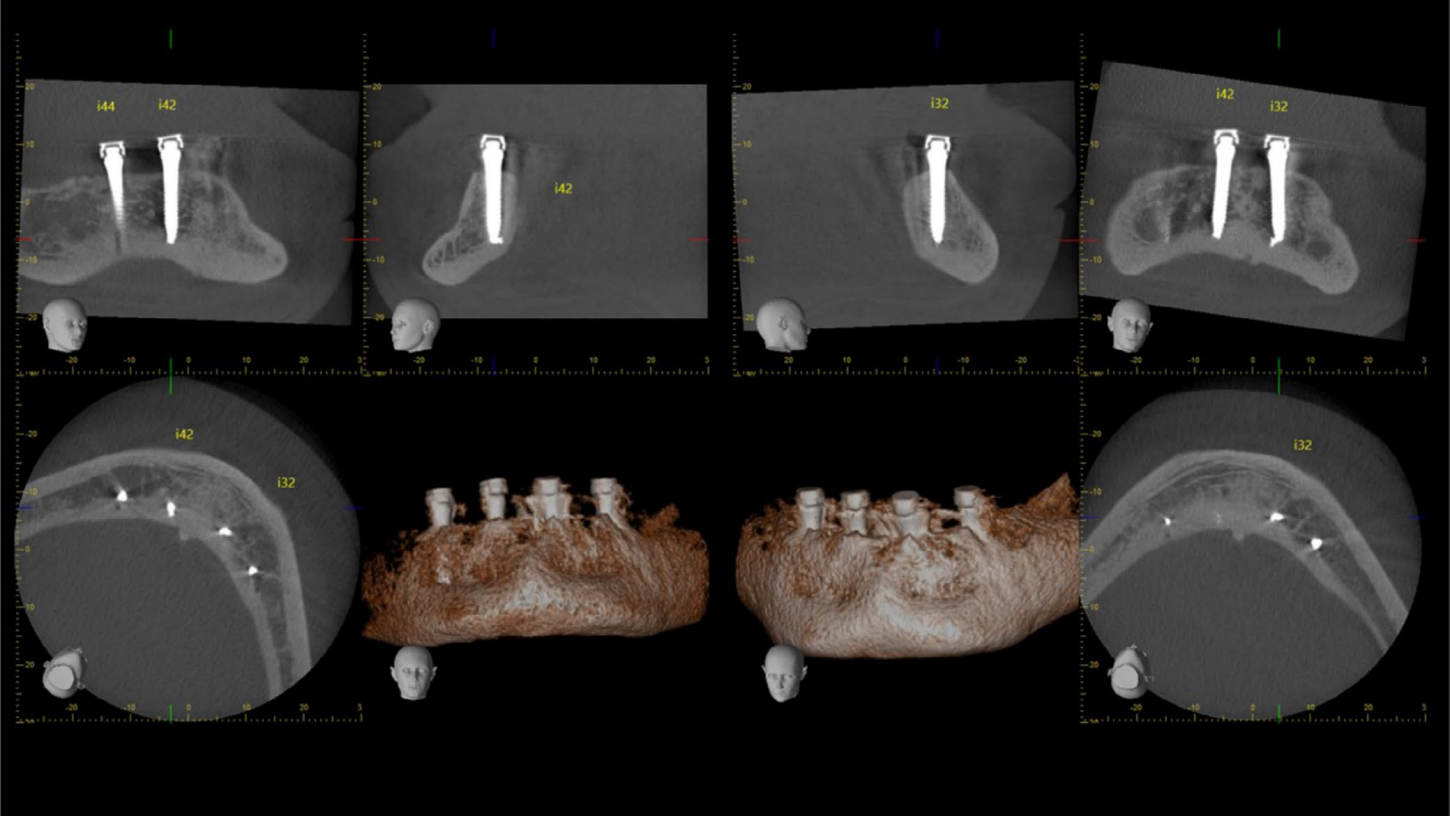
CBCT exam of a clinical case with apical implant fractures detected in post‐insertion assessments.
